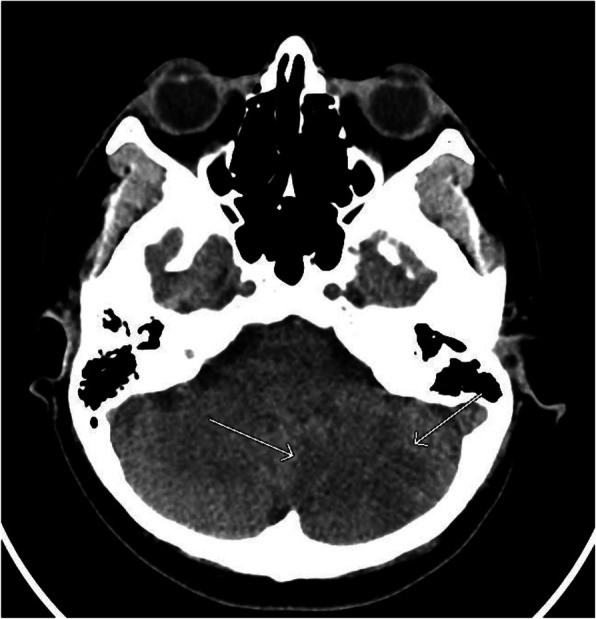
Non-contrast CT scan of the head demonstrates a probable acute or subacute infarction in the left cerebellar hemisphere
